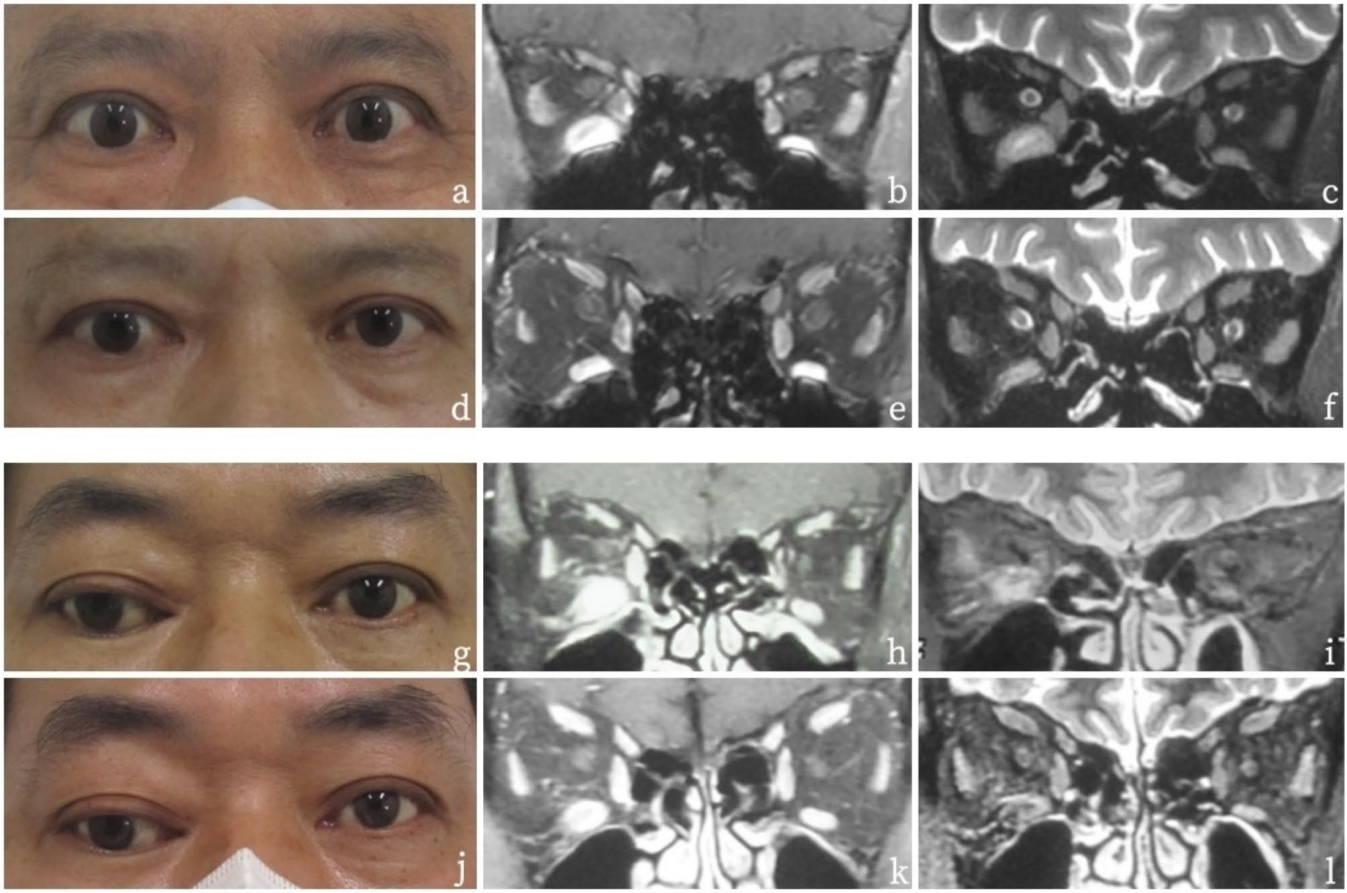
Legends, (a–f): A TED patient with no significant abnormality in ocular appearance, presenting only with limited eye movement. MRI revealed active extraocular muscles. (a–c): Images at the initial visit; (d–f): Images at three months post-IMRT. (g–l): A TED patient with pronounced restrictive strabismus. MRI showed active extraocular muscles. (g–i): Images at the initial visit; (j-l): Images at three months post-IMRT. (a, d, g, j): Ocular appearance; (b, e, h, k): T1-weighted orbital enhanced MRI images; (c, f, i, l): T2-weighted orbital enhanced MRI images.
